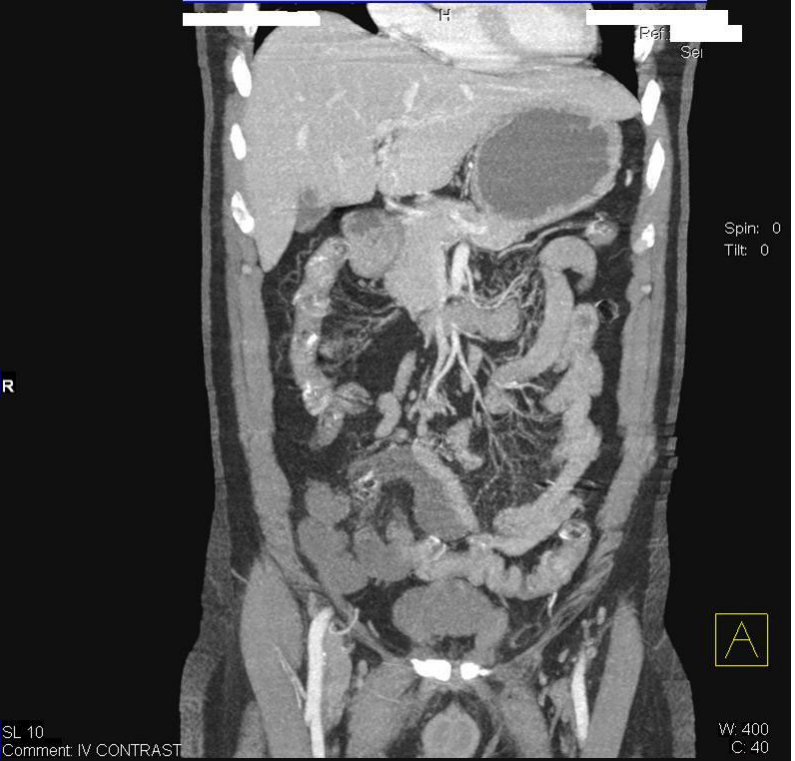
Abdominal Angio CT coronal reconstructed image revealed; marked discrepancy of the bowel wall enhancement between the distal ileum and right colon, and the proximal ileal loops and left colon, denoting arterial ischemia.
